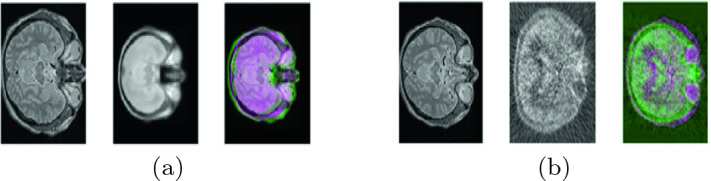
MRI/X brain image registration: (a) mono-modal MRI/MRI atlas (from left to right: MRI image, MRI atlas and superposed images), (b) multi-modal MRI/PET (from left to right: MRI image, PET image and superposed images).
